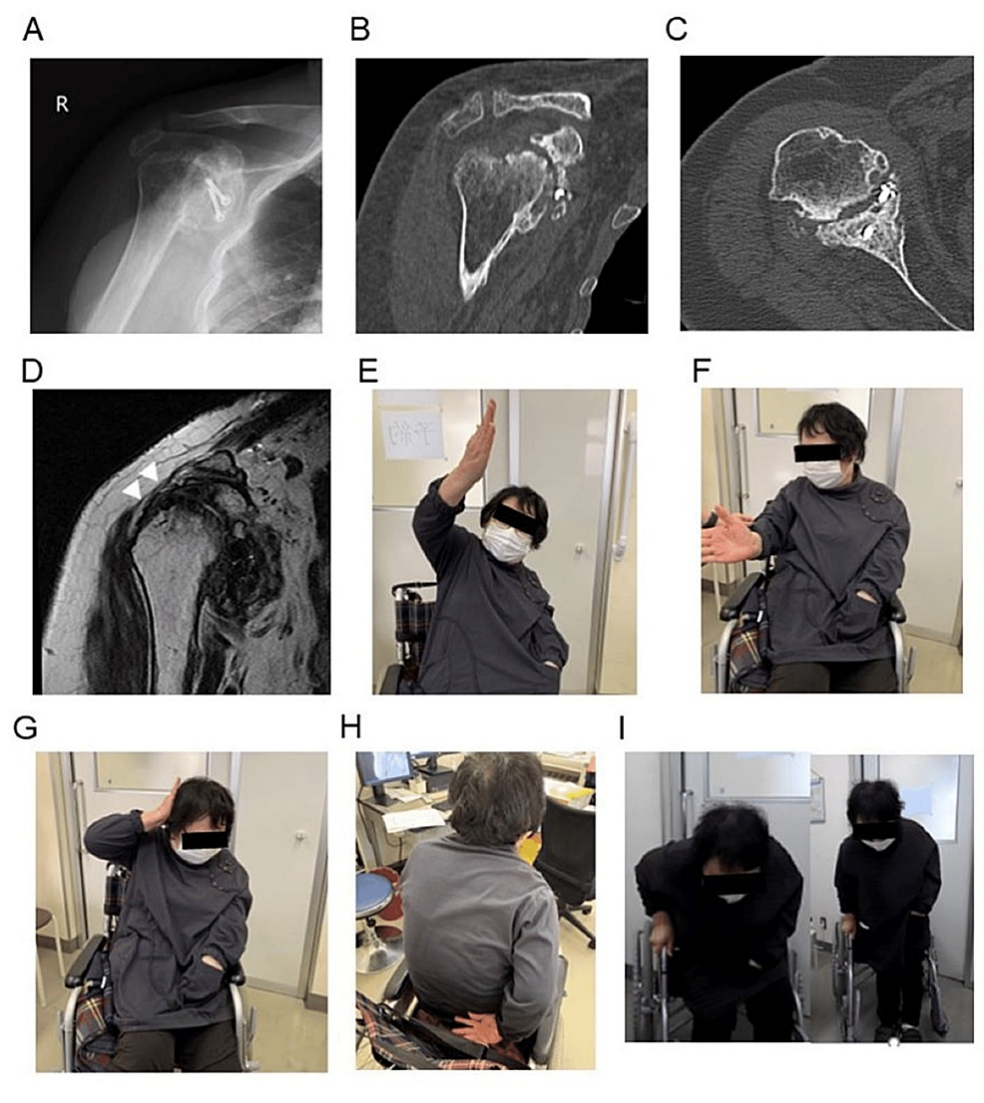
Fifty months after surgery. A) Radiograph showing progression of the osteoarthritis. B, C) CT scans showing reconstruction of the glenoid surface by the transferred coracoid. D) White arrowheads indicate integrity of the repaired supraspinatus tendon on T2-weighted MRI. E) Flexion. F) External rotation. G) The patient can touch the back of her head with the elbow back. H) Internal rotation. I) The patient can stand up by herself.
